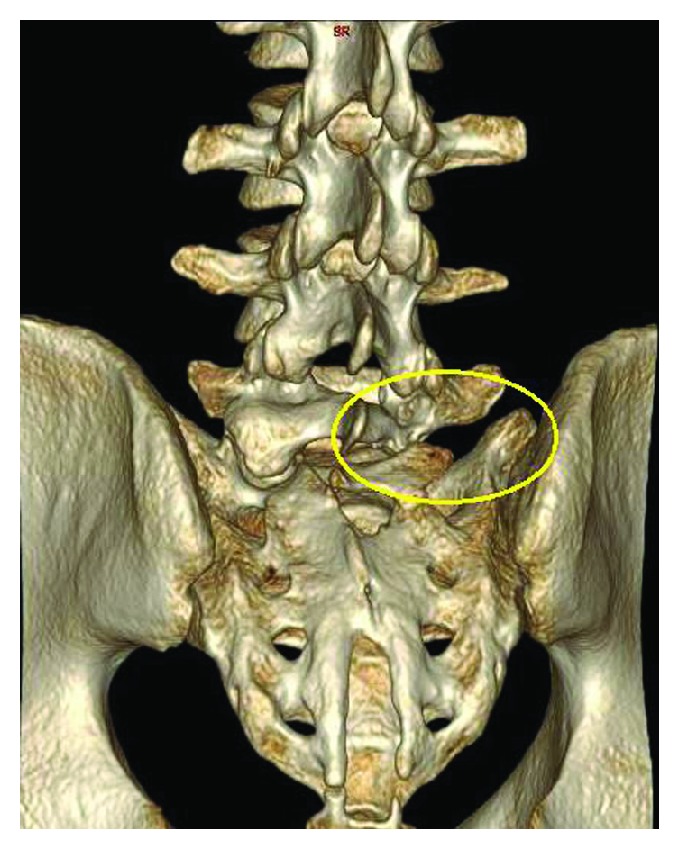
CT scan 3D reconstruction shows agenesis of the L5 inferior articular process on the right side with no other related bony abnormalities identified.
